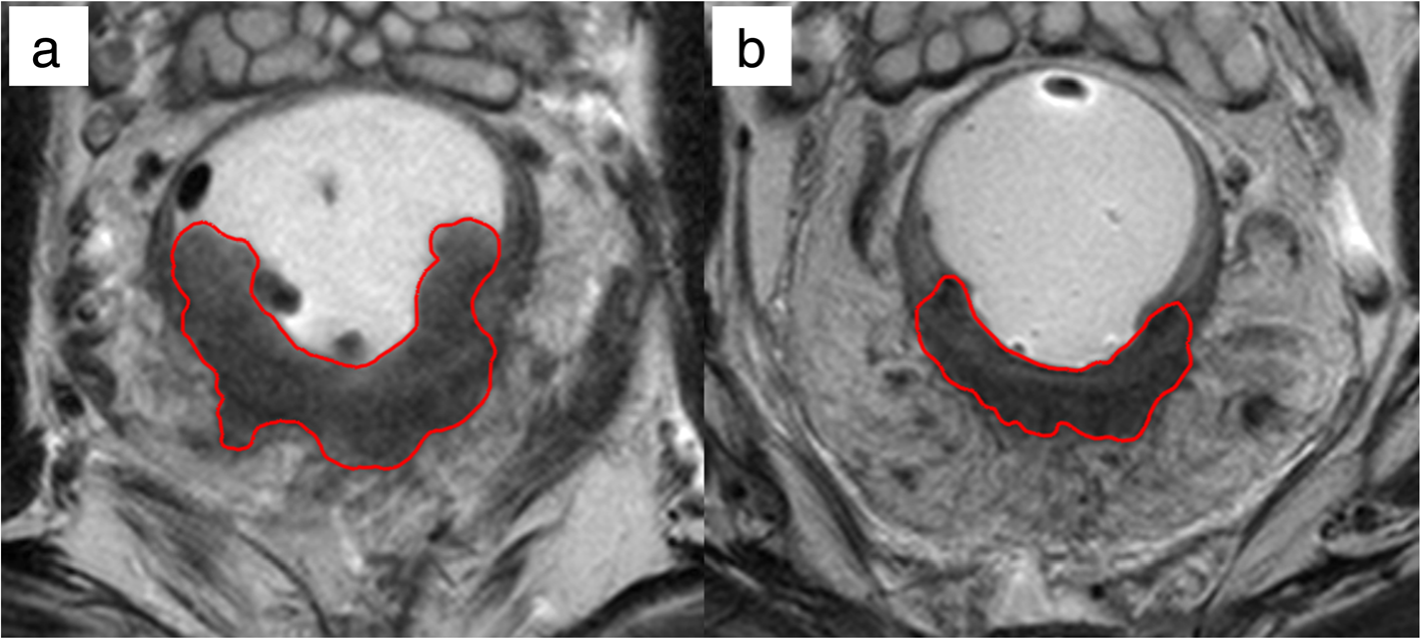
Examples of tumor segmentation on MRI acquired (a) before and (b) after preoperative CCRT
